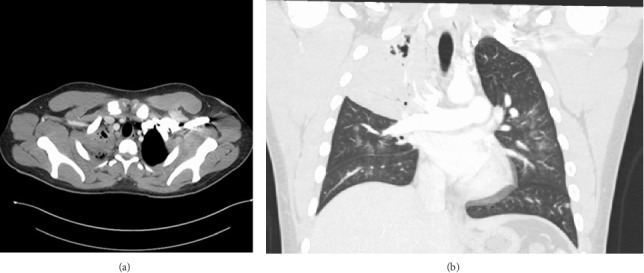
CT chest imaging. Consolidative opacities with cavitation seen throughout the right upper lobe in (a) the axial section and (b) the coronal section.
